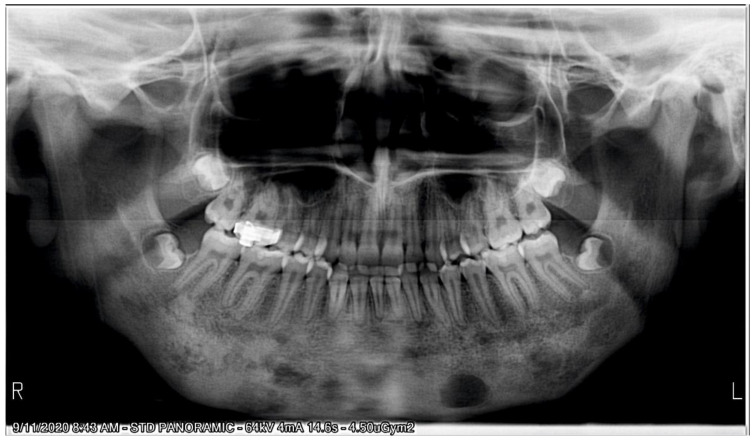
Panoramic radiograph at the one-year follow-up appointment.
